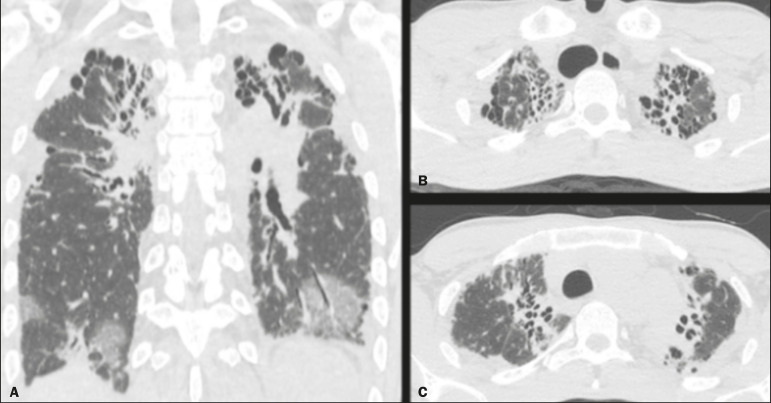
Coronal CT slice (A) and axial CT slices (B,C), showing signs of architectural distortion, characterized by volume reduction and retractile opacities, together with bilateral cylindrical and varicose bronchiectasis, predominantly in the upper lung fields, in a patient who had undergone chemotherapy and radiotherapy 20 years prior for the treatment of lymphoma.
